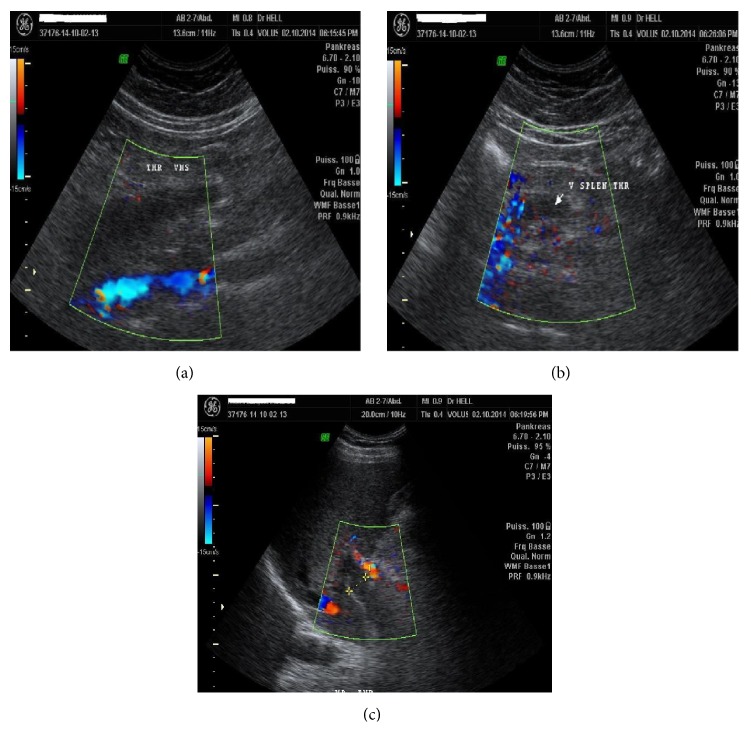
Doppler ultrasonography: showing in (a) the superior mesenteric vein thrombosis, in (b) the splenic vein thrombosis, and in (c) the portal vein thrombosis. With the permission of Medical Imaging Centre (MIC) in Yaoundé.
